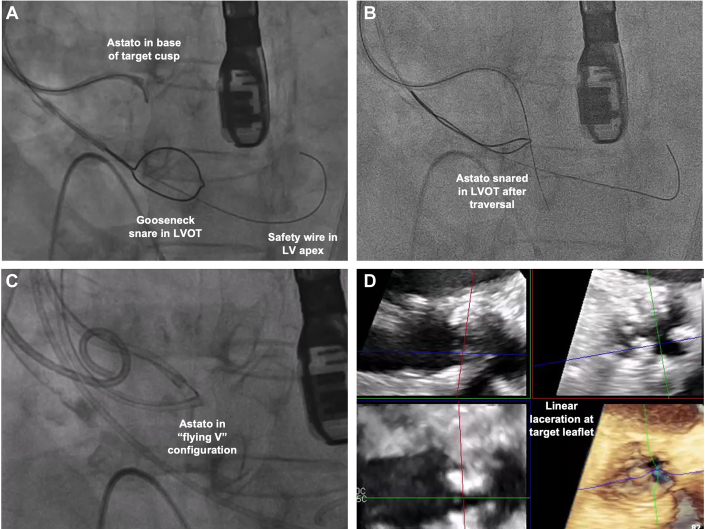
BASILICA Technique (A) Astato guidewire directed toward the base of the target leaflet. Gooseneck snare in the LVOT. Safety wire in the LV apex. (B) After traversal of the target leaflet, Astato guidewire is snared in the LVOT. (C) A "flying V" configuration is created in the Astato wire by denuding and kinking a segment near the end of a wire converter, such as PiggyBack wire converter (Teleflex) or 0.035-inch microcatheter. (D) Echocardiography showing linear laceration at the target leaflet. Abbreviations as in Figure 1.
